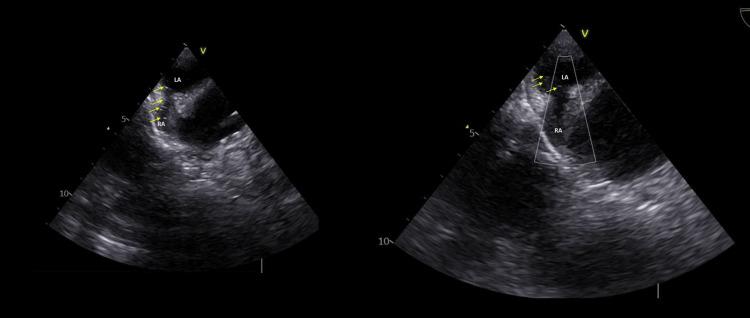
Two-dimensional echocardiogram with agitated saline contrast. The yellow arrows indicate the passage of agitated saline microbubbles across the patent foramen ovale.
